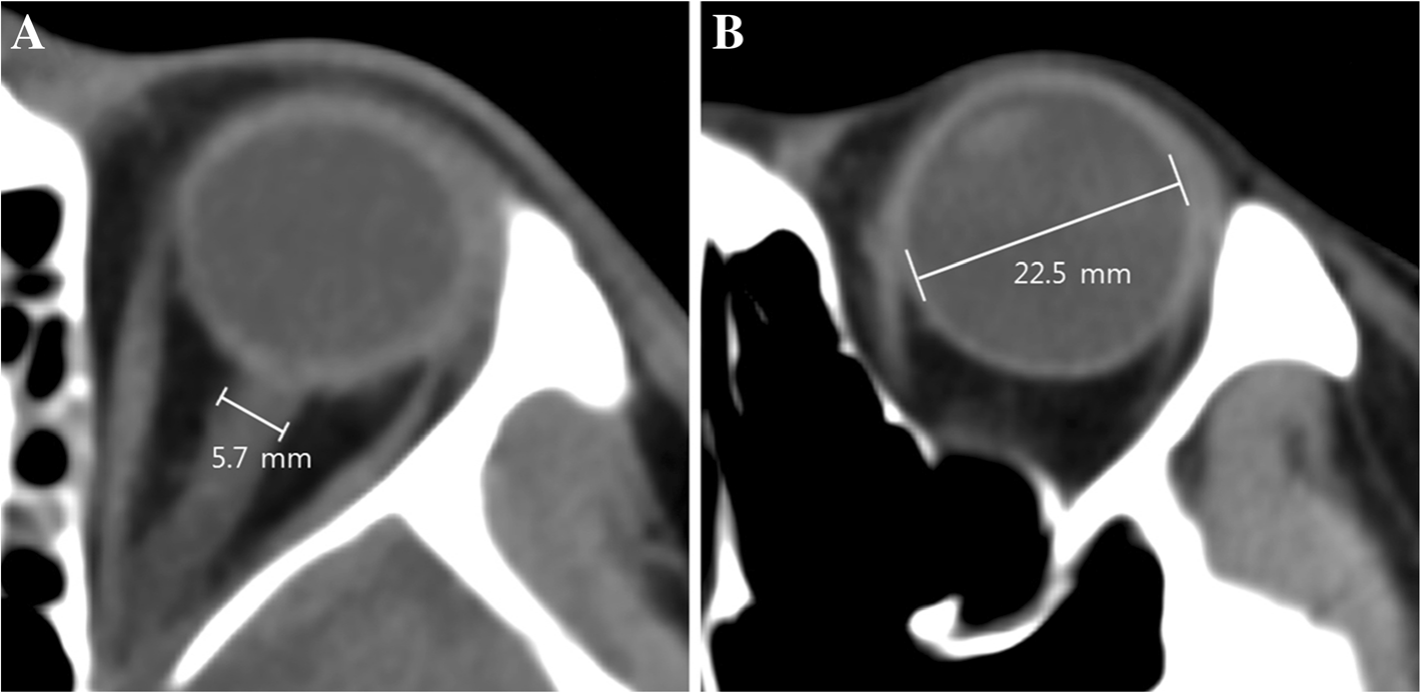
Measurement of optic nerve sheath diameter (a) and eyeball transverse diameter (b) on the brain computed tomography scan
